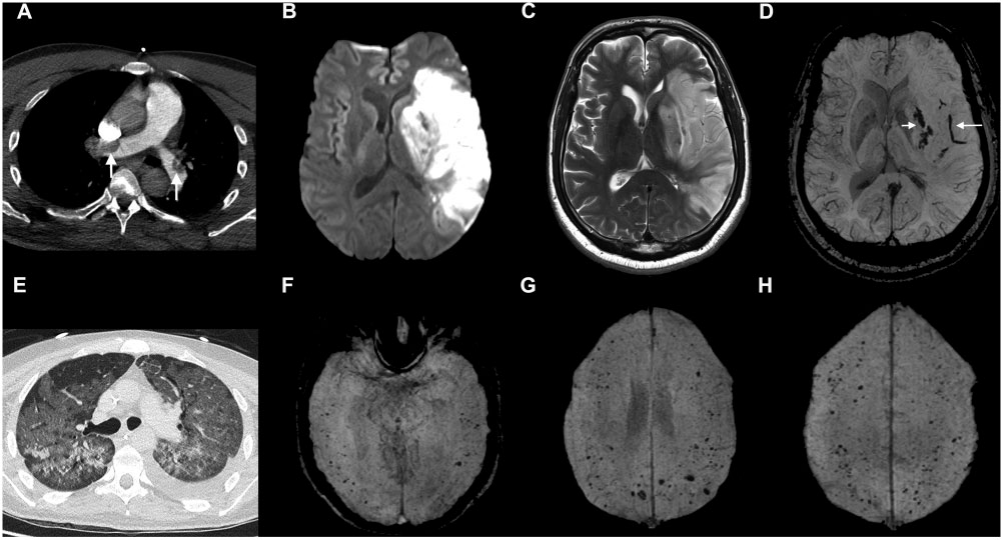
Imaging from Patient 27, with cerebral infarction and pulmonary thromboembolism (A–D), and Patient 41, with microhaemorrhages (E–H). (A–D) Patient 27: CT pulmonary angiogram (A) demonstrated large emboli in the right and left pulmonary arteries (arrows). DWI (B), T_2_-weighted FSE (C) and SWI (D) images show restricted diffusion (B) and T_2_ hyperintensity (C) in the left basal ganglia and cortical territory of left middle cerebral artery. The SWI image (D) shows haemorrhagic transformation in the basal ganglia (short arrow) and a long intravascular thrombus in a Sylvain branch of the left middle cerebral artery (long arrow). (E–H) Patient 41: chest CT (E) shows severe COVID-19 pneumonitis. SWI images (F–H) demonstrate numerous cerebral microbleeds in the temporal, frontal and parietal lobes, predominantly located at the grey/white matter junction.
